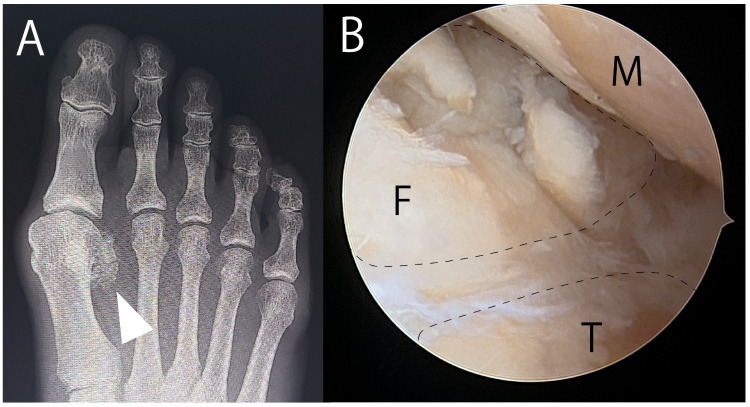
Fragmented avascular necrosis of the fibular sesamoid treated with arthroscopic sesamoidectomy. (A) Radiograph showing avascular necrosis of the lateral sesamoid with fragmentation (arrowhead). (B) Arthroscopic view showing fragmentation of the fibular sesamoid (F). This case was treated with arthroscopic sesamoidectomy. Dotted circles indicate the tibial and fibular sesamoids. F, fibular sesamoid; M, metatarsal head; T, tibial sesamoid.
